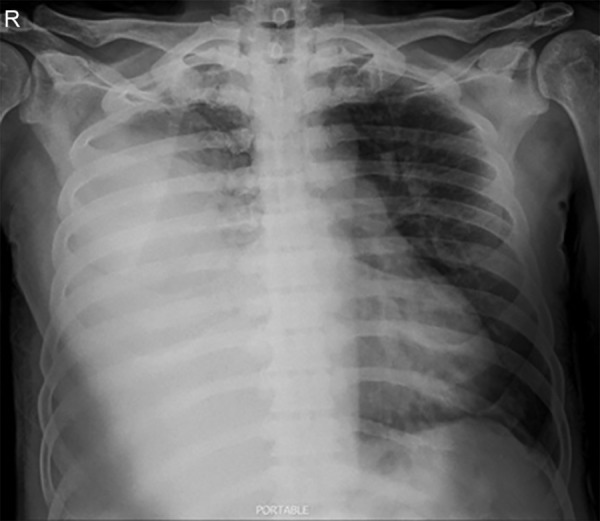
Chest X-ray PA view showing the massive right sided pleural effusion
